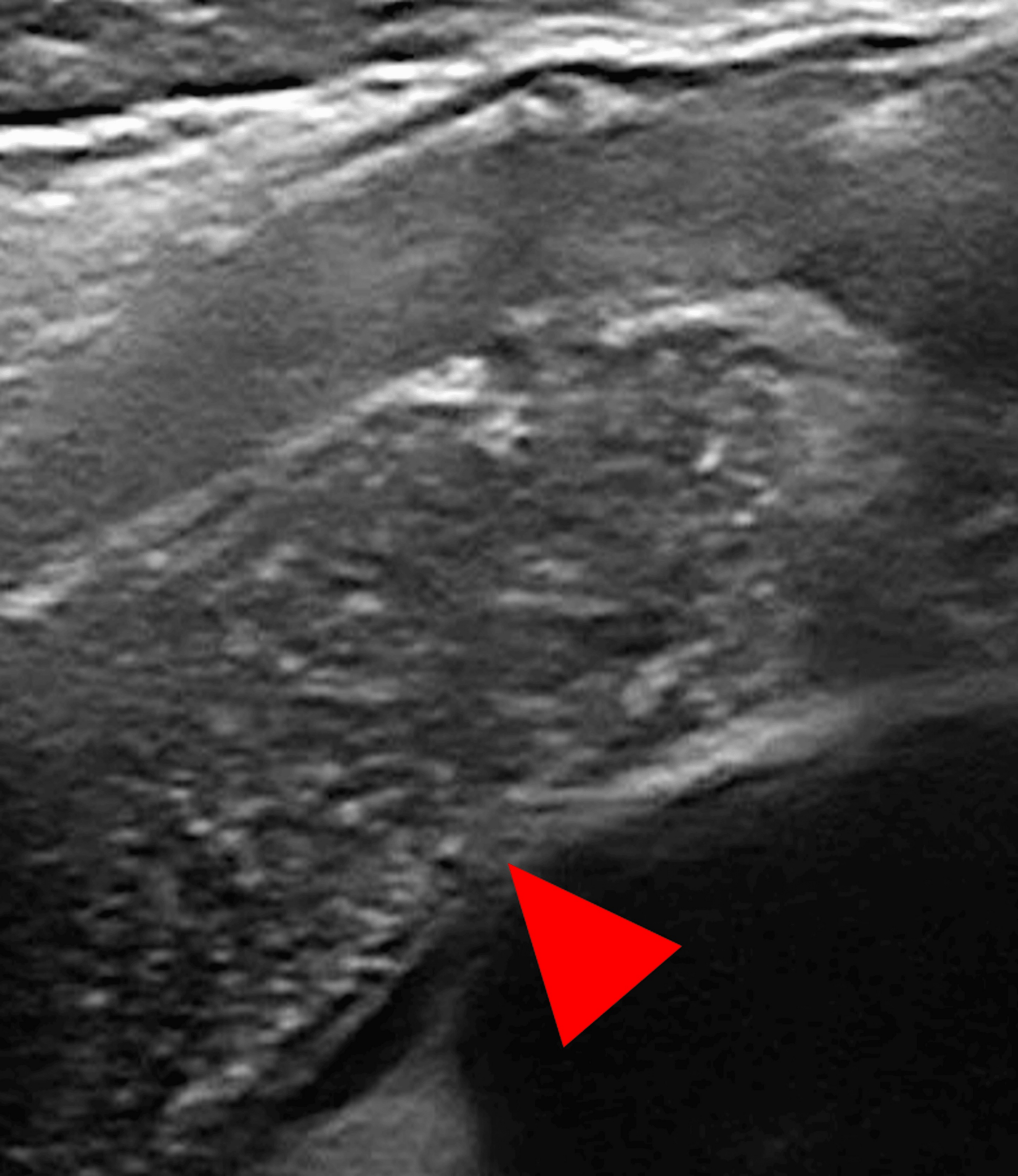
Ultrasound image of the thymus Chest ultrasound image of the normal thymus with an identical internal pattern (red arrowhead).
